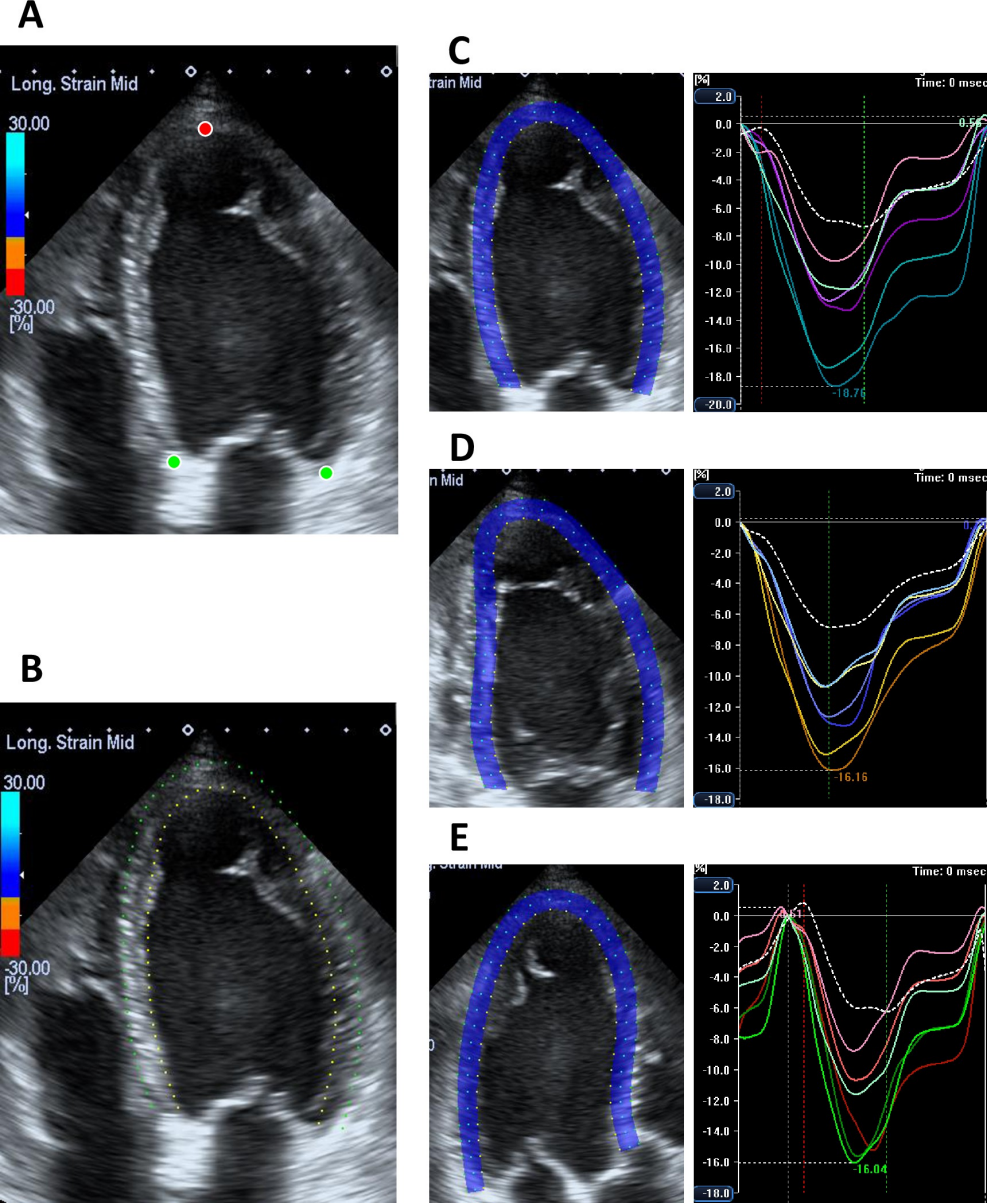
Process of semiautomated layer specific speckle tracking analysis. (A) Apical 4-chamber view at the end-diastole; 3 points (both side of the mitral annulus [green dots] and apex [red dot]) are manually determined. (B) Automatic determination of the endocardial borders (endocardial border [yellow dots] and epicardial border [green dots]. (C) Layer strain analysis on the apical 4-chamber view; blue dots represent mid-myocardial tracking line. The right panel shows segmental strain curve. (D) Layer strain analysis on the apical 2-chamber view. (E) Layer strain analysis on the apical long-axis view.
